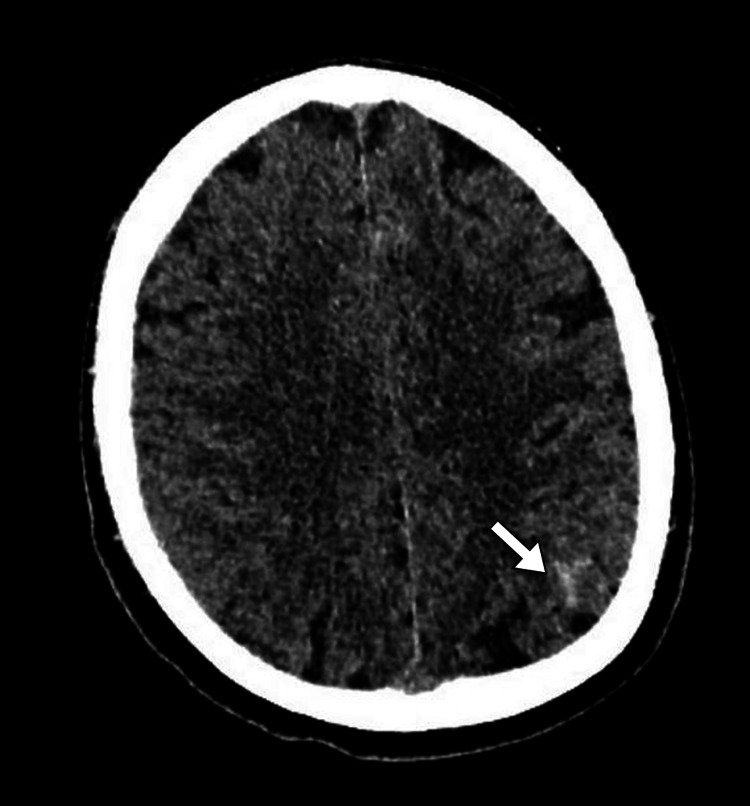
Axial head CT image shows subarachnoid hemorrhage (arrow) in the left parietal lobe CT: computed tomography
